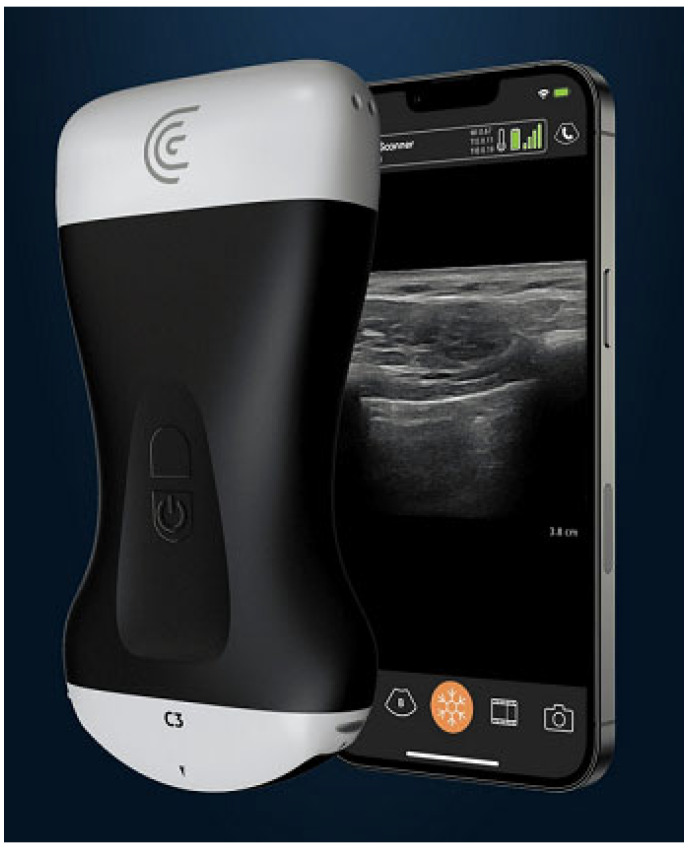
Image of ultrasound probe with linked mobile device onto which image is transmitted. There are a number of such devices, some with wire-to-the-phone, and others with Bluetooth^®^ or Wi-Fi connectivity.
